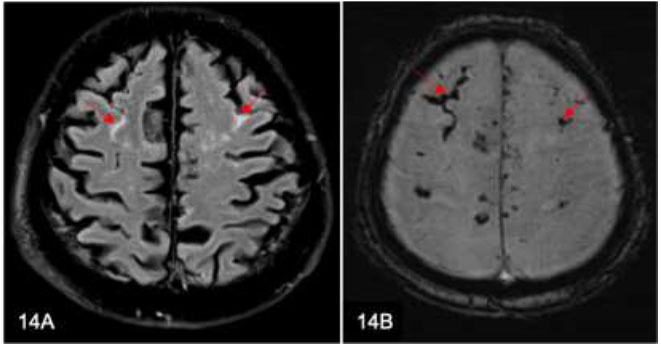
A 72-year-old man sustained an acute subarachnoid hemorrhage (SAH) following a fall incident. Axial FLAIR image (A) demonstrates hyperintensity along bilateral cerebral sulci, while Susceptibility-weighted imaging (B) demonstrates corresponding susceptibility (arrows), indicating SAH. These features masquerade as leptomeningeal disease.
